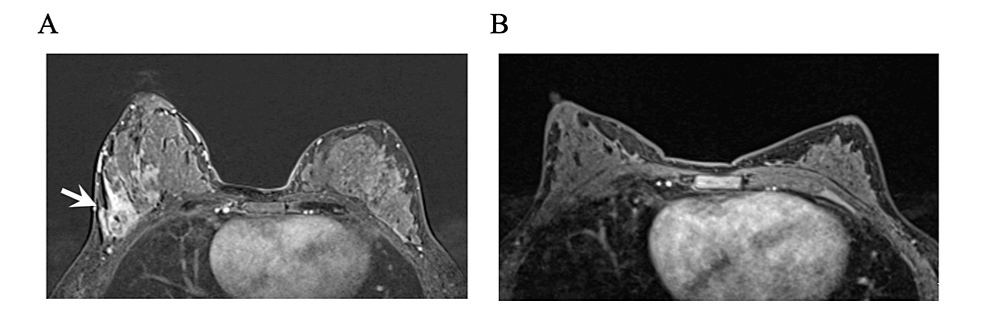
Bilateral breast MRI. Contrast-enhancing 5.8 cm mass in the upper outer quadrant of the right breast (white arrow) in the background of enlarged, enhancing, and increased fibroglandular breast tissue from breastfeeding (A), and complete radiological response in the right breast and axilla obtained post-treatment with no areas of mass or contrast enhancement present (B).
